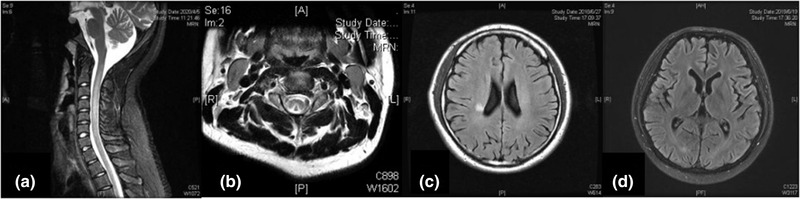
Representative magnetic resonance (MR) images of central nervous system abnormalities in patients abusing nitrous oxide (N_2_O). (a) MR image of cervical vertebrae showing a high T2 signal. (b) T2‐weighted image from cervical MRI showing an inverted “V” sign in the posterior funiculus of the spinal cord. (c) magnetic resonance imaging (MRI) of the skull showing a T2FLAIR hyperintense signal in the right radiation coronal area. (d) Brain MRI showing brain atrophy
